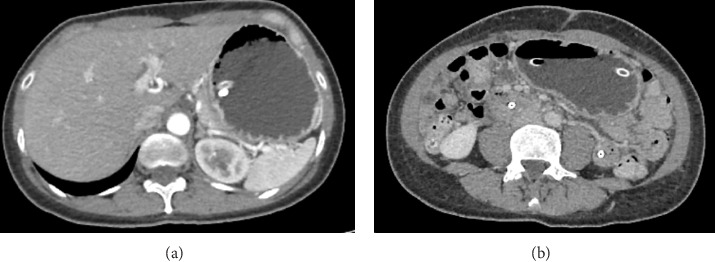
(a) Axial contrast-enhanced CT image showing marked gastric distension with an abrupt transition at the pyloric region. The gastric outflow tract narrows sharply into a collapsed bowel segment, consistent with gastric outlet obstruction. (b) Axial contrast-enhanced CT image of the mid-abdomen, demonstrating prominent gastric distension and multiple collapsed small bowel loops throughout the abdomen, consistent with a gastric outlet obstruction pattern.
